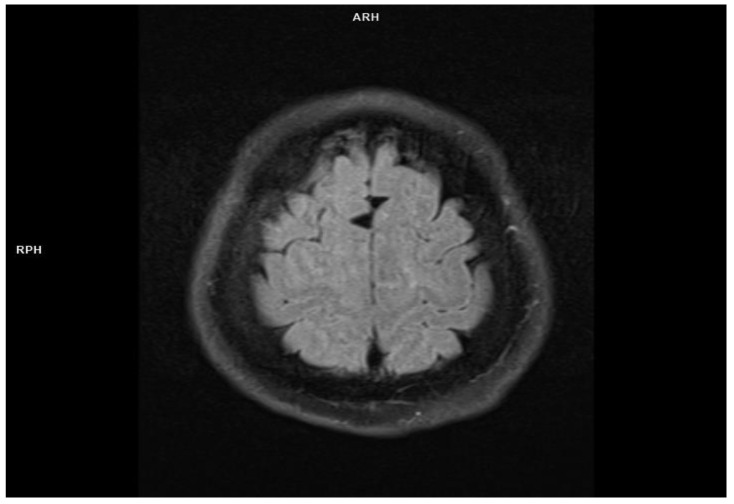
Bilateral shallowed sulci on the convexities of the brain—Flair sequence (MRI imaging).
